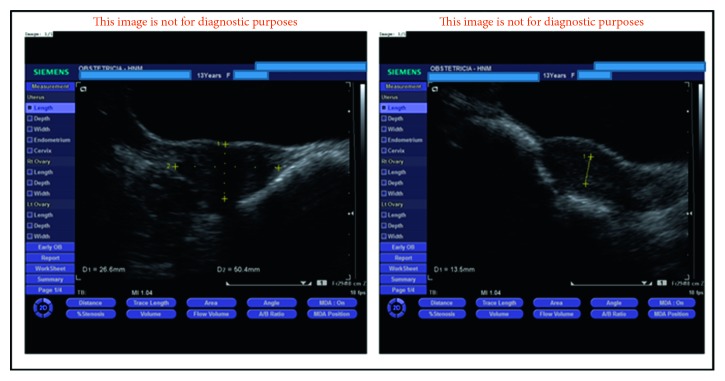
Ultrasound images showing a normal size uterus with two endometrial cavities both with a regular endometrium.
